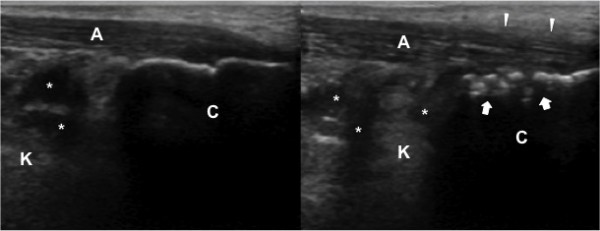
A 13-year-old boy with bilateral heel pain who was eventually diagnosed as enthesitis-related arthritis. Comparative ultrasound evaluations (longitudinal view) demonstrate increased thickness, and edema of the right achilles tendon (A). The paratenon is blurred *(white arrow heads) *and there are irregularities *(white arrows) *at the insertion site on calcaneus (C) (right image). The echogenicity of Kager's fat pad (K) is also irregular on both sides.
